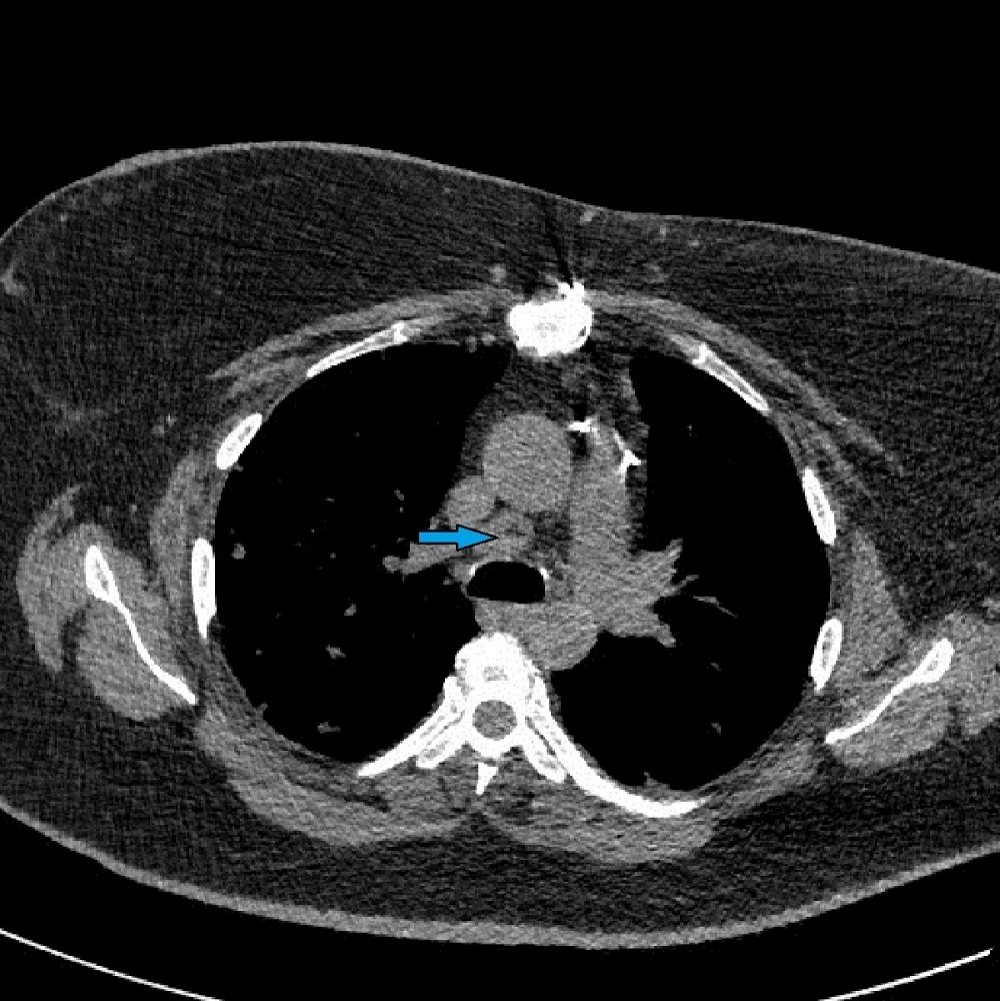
Computed tomography of the chest with mediastinal window showing an enlarged mediastinal lymph node
